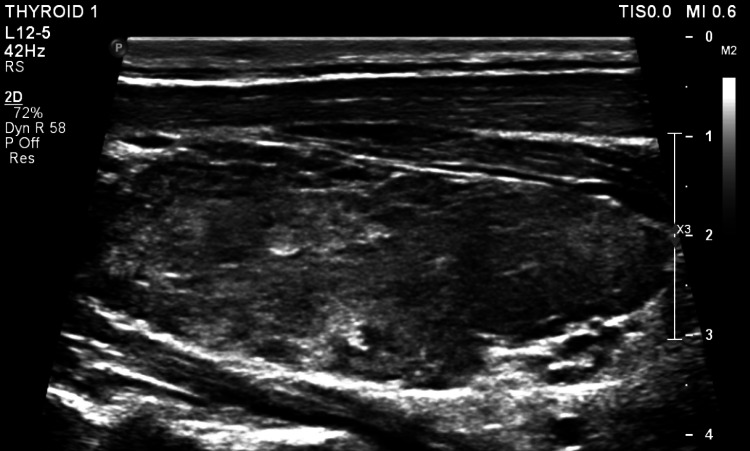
Patient's thyroid ultrasound shows thyroid tissue with hyperechoic nodules of various sizes consistent with Hashimoto's thyroiditis.
